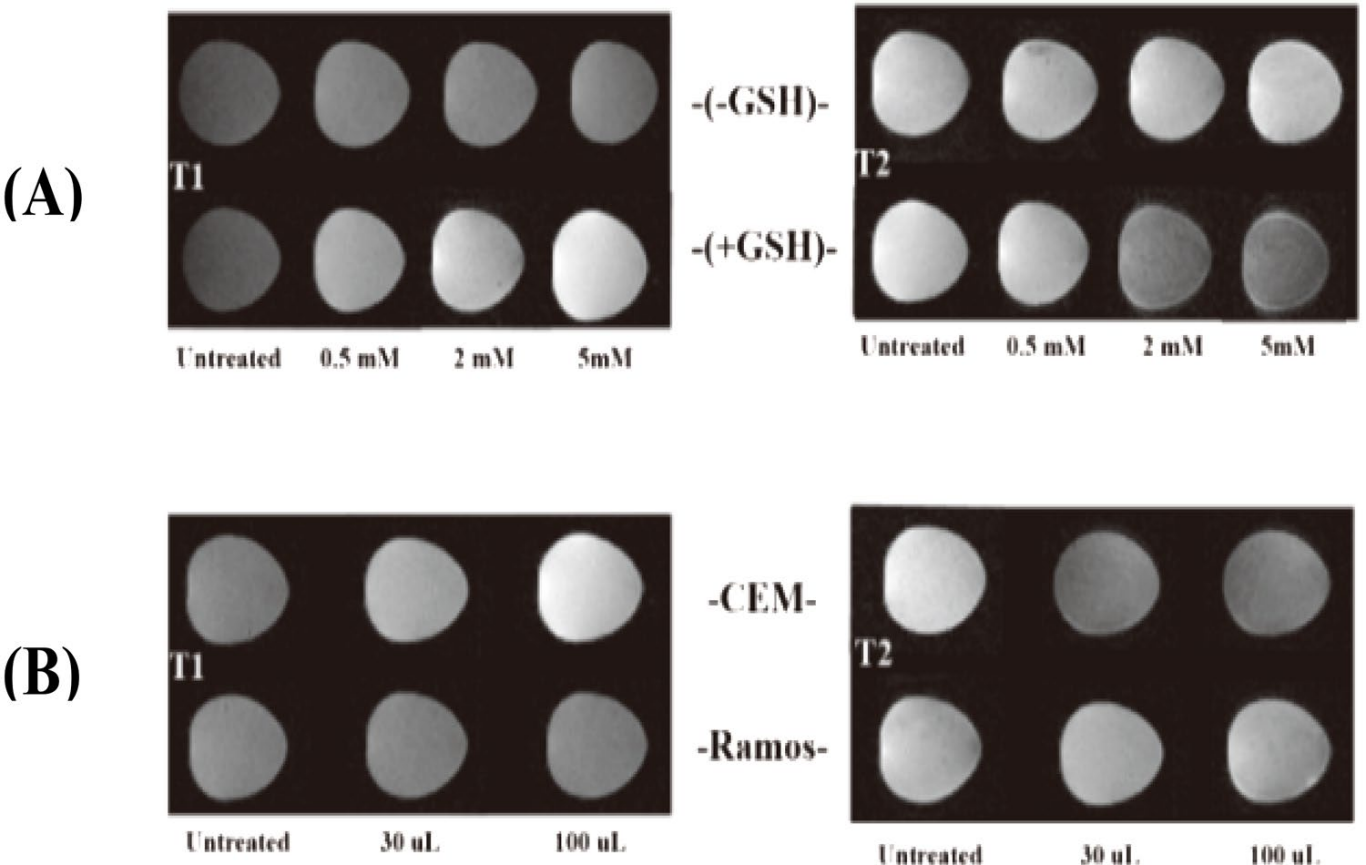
(A) T1-weighted and T2-weighted MRI images of MnO_2_ nanoprobes solution treated with GSH. (B) T1-weighted and T2-weighted MRI images of CCRF-CEM cells and Ramos cells incubated with MnO_2_ nanoprobes at various volume.
